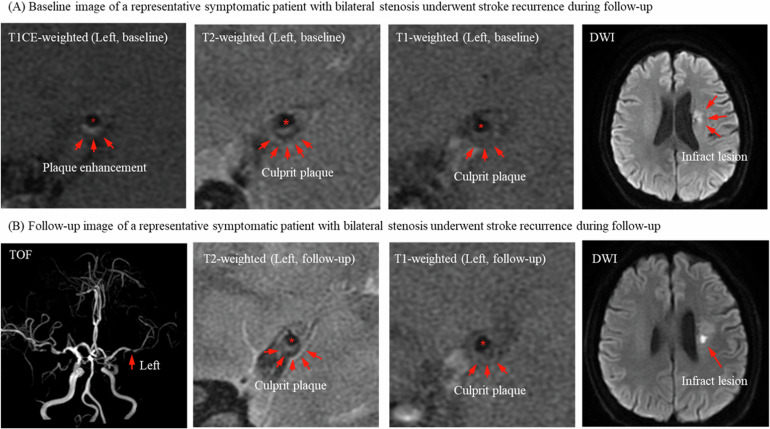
Baseline and follow-up high-resolution magnetic resonance imaging (hrMRI) of a patient with recurrent ischemic stroke on the same territory. A 60-year-old male patient with ischemic stroke underwent hrMRI examination upon admission. TOF-MRA found bilateral middle cerebral artery (MCA) stenosis and left MCA was regarded as the culprit lesion. The images demonstrate the appearance of culprit plaque in the left hemisphere on black blood 2-dimensional T2, and T1 sequences (red arrows). The diffusion-weighted imaging (DWI) sequence shows cerebral infarction within the left MCA supply area (red arrow). B The patient experienced symptomatic recurrence and was readmitted 2 months after discharge for further hrVWI examination, which confirmed recurrent ischemic stroke within the same territory. TOF-MRA and Black blood T2-weighted imaging and T1 sequences show the progression of stenosis and plaque burden compared to baseline images (red arrow), while the DWI sequence demonstrates a new ischemic lesion (red arrow). *Lumen
